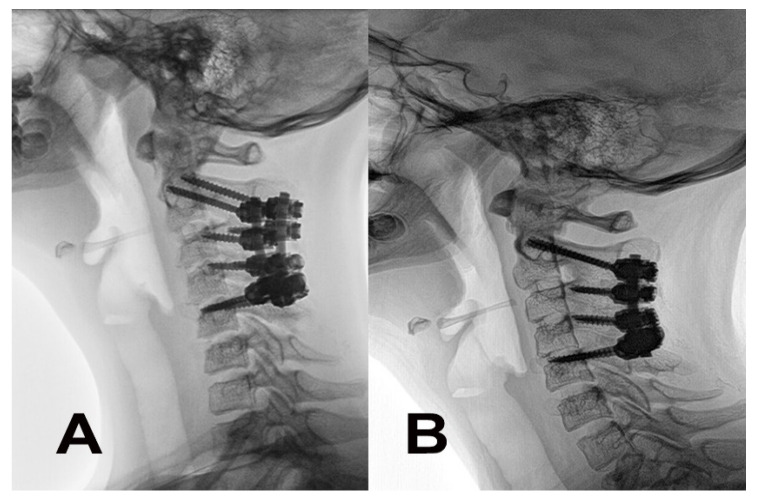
Evolution of the cervical curve after the corrective posterior surgery and fusion. (A) Radiography performed immediately after the surgery revealed a correction to a flat, straight curve. (B) Restoration of the physiological curve after 3 years.
